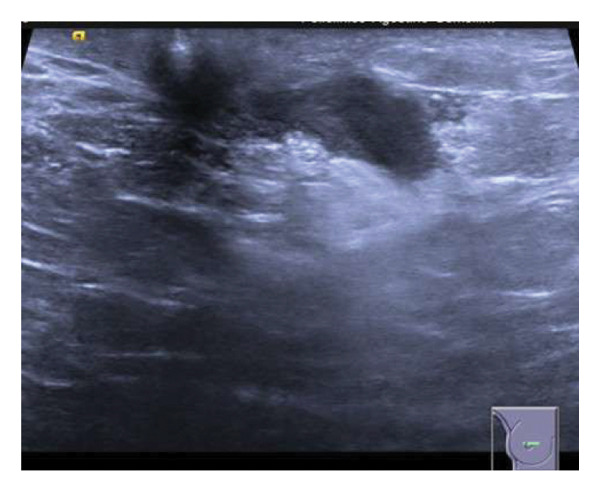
A 36‐year‐old woman presented with right breast pain during breastfeeding. Ultrasound reveals a retro areolar nodular lesion with irregular margins and hypoechoic appearance, initially suggestive of a breast abscess.
